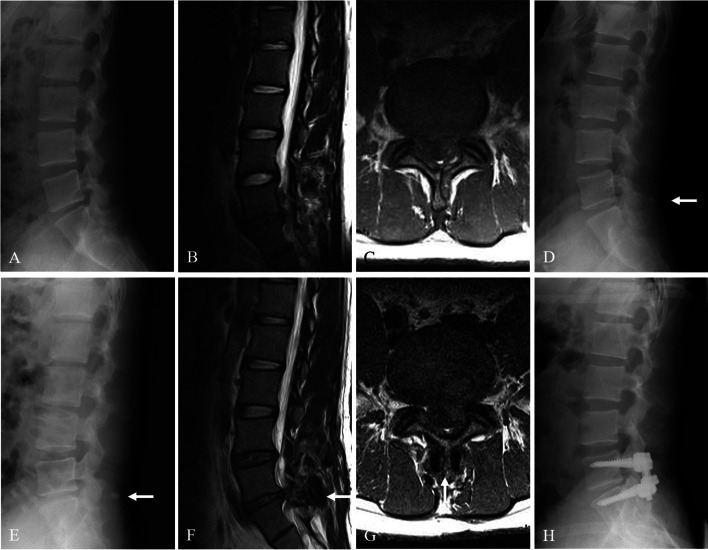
A 27-year-old man with lumbar disc herniation (LDH) at L5–6. Lateral (A) radiographs showing mild degenerative changes at L5–6. Magnetic resonance images (B and C) showing LDH at L5–6. The patient underwent discectomy and DIAM implantation at L5–6 (white arrow) (D). At 5 years after surgery, lateral radiograph (E) and magnetic resonance images (F and G) show maintenance of DIAM (white arrows) but recurrent LDH and stenosis at L5–6. The patient underwent DIAM removal, total laminectomy, another discectomy, and fusion at L5–6 (H)
